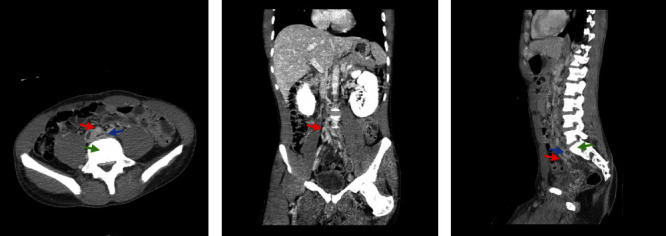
(a) CT abdomen/pelvis with contrast (axial view) showing obstructed vein (blue arrow) in between artery (red arrow) and vertebrae (green arrow). (b) CT abdomen/pelvis with contrast (coronal view) showing site of obstruction at left common iliac vein crossing in between right common iliac artery and L5 vertebrae. (c) CT abdomen/pelvis with contrast (lateral view) showing obstructed vein (blue arrow) in between artery (red arrow) and vertebrae (green arrow).
